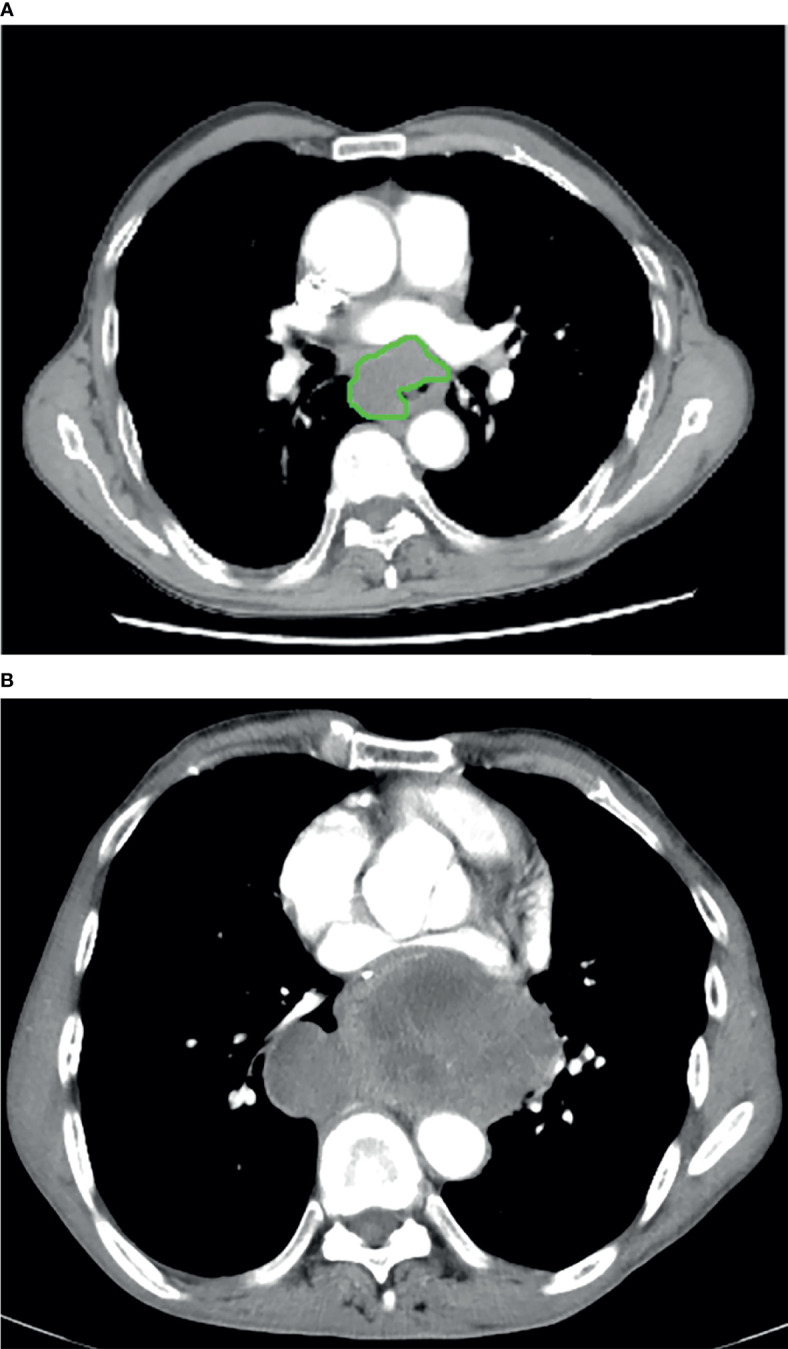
In a 61-year-old male with esophageal squamous cell carcinoma at cT_2_N_0_M_0_, the preoperative thoracic contrast-enhanced CT scans depict the gross tumor volume obtained by manual delineation along the margin of the abnormal esophageal wall (A) slice-by-slice, and the gross tumor volume is 58.66 cm^3^. Regional and local recurrence has been found 12 months after radical esophagectomy during the follow-up period (B).
